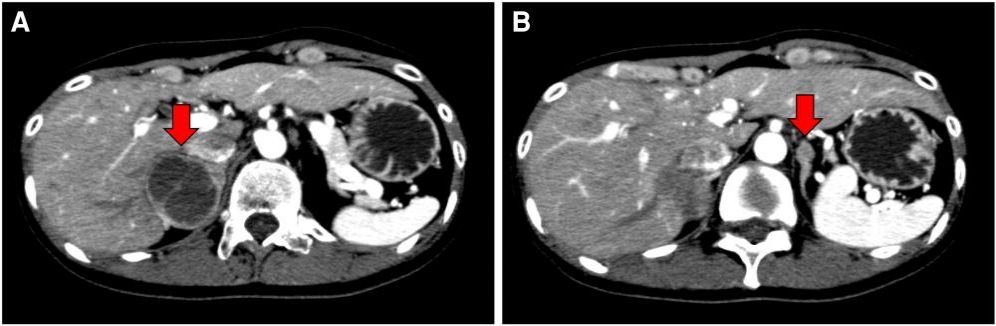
CT findings. A 4.6 × 3.8 cm mass with cystic changes was observed in the right adrenal gland (A), and a 1.2 × 1.2 cm solid mass was observed in the left adrenal gland (B).
